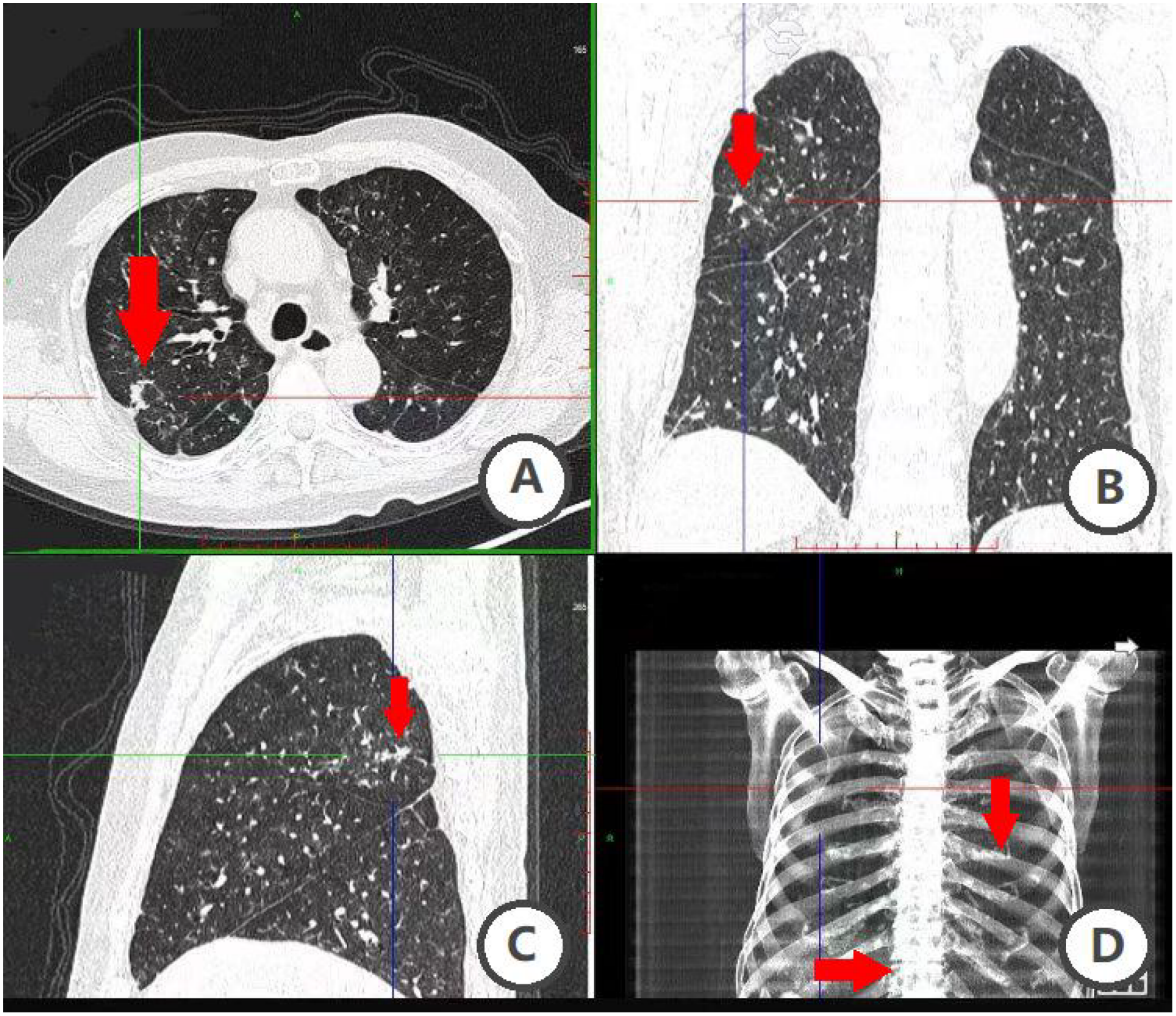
Unenhanced CT combined with 3D post-processing. (A–D) figure suggests that the patient has multiple lung nodules and metastasis is possible; uneven bone density of ribs and thoracic vertebrae.
